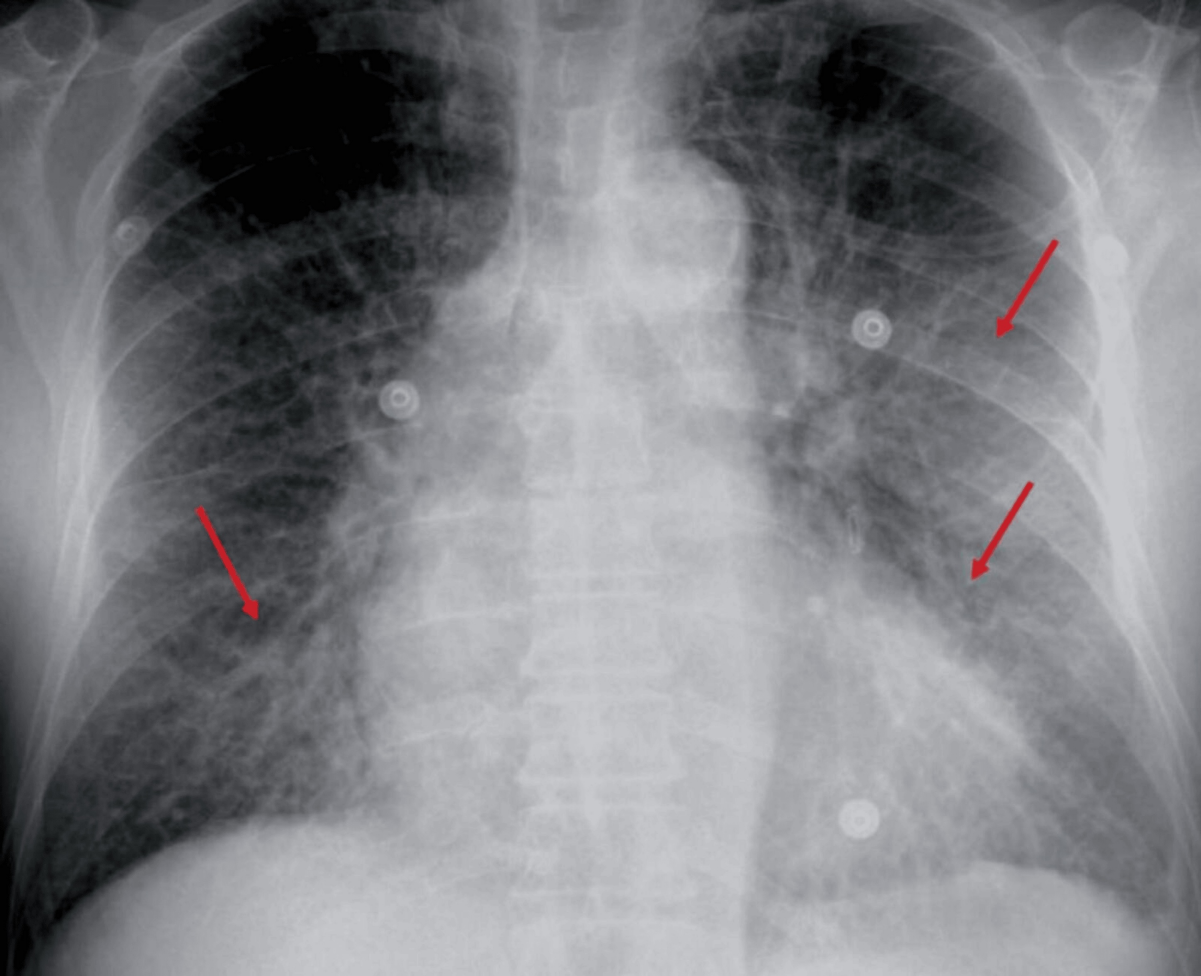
Chest radiograph demonstrating pulmonary congestion and bilateral pleural effusions Chest radiograph demonstrating pulmonary vascular congestion (red arrows) and bilateral pleural effusions. In the clinical context of peripheral edema and markedly elevated NT-proBNP levels, these findings support the diagnosis of acute ischemic heart failure.
